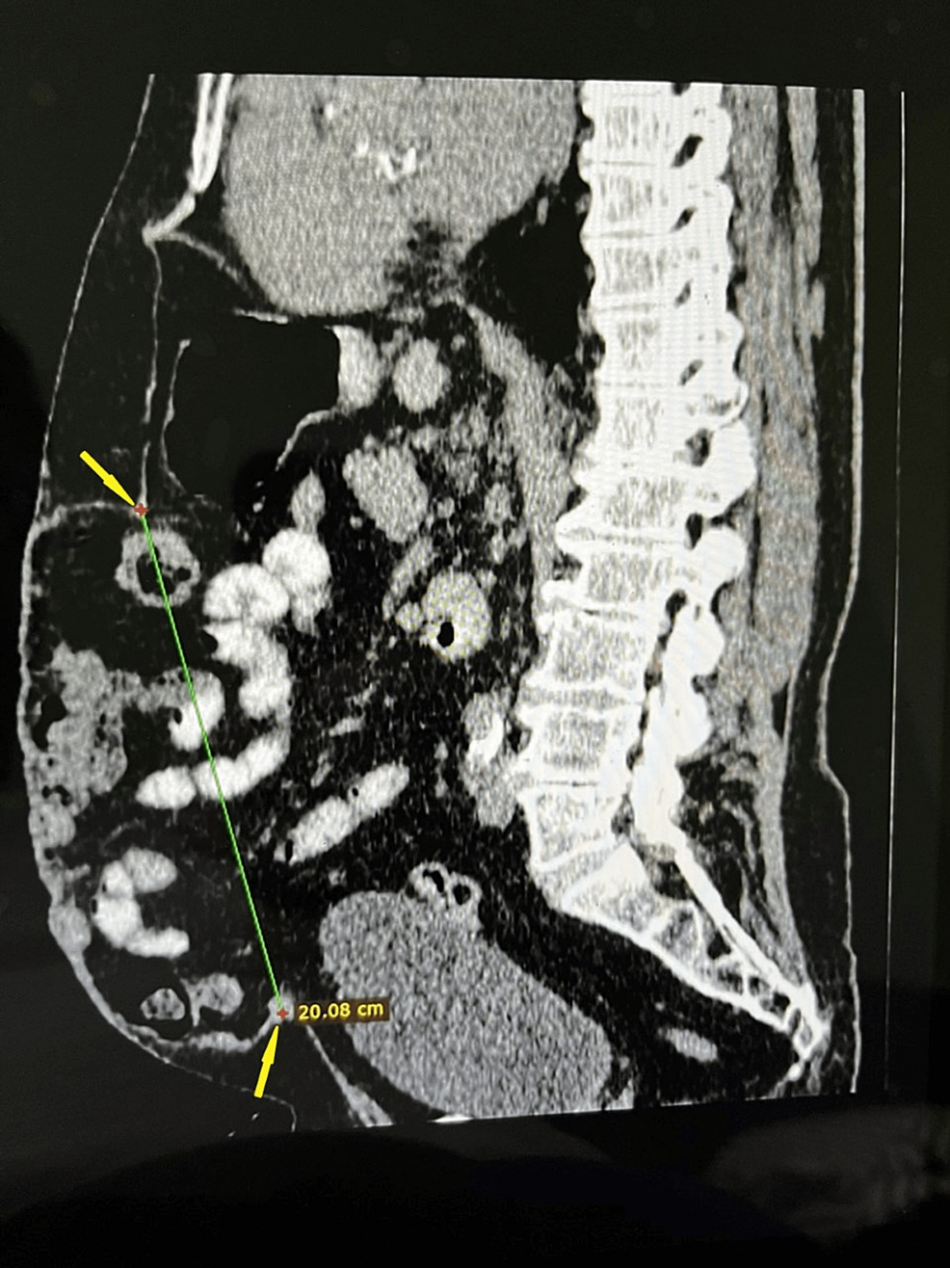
Preoperative sagittal abdomen CT scan with a 20-cm long midline defect CT: computed tomography
